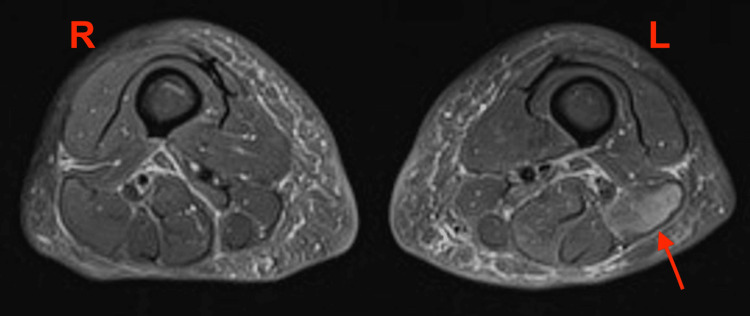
Axial STIR MRI image of both thighs demonstrating a high signal in the biceps femoris on the left suggesting localized myositis STIR: Short tau inversion recovery
